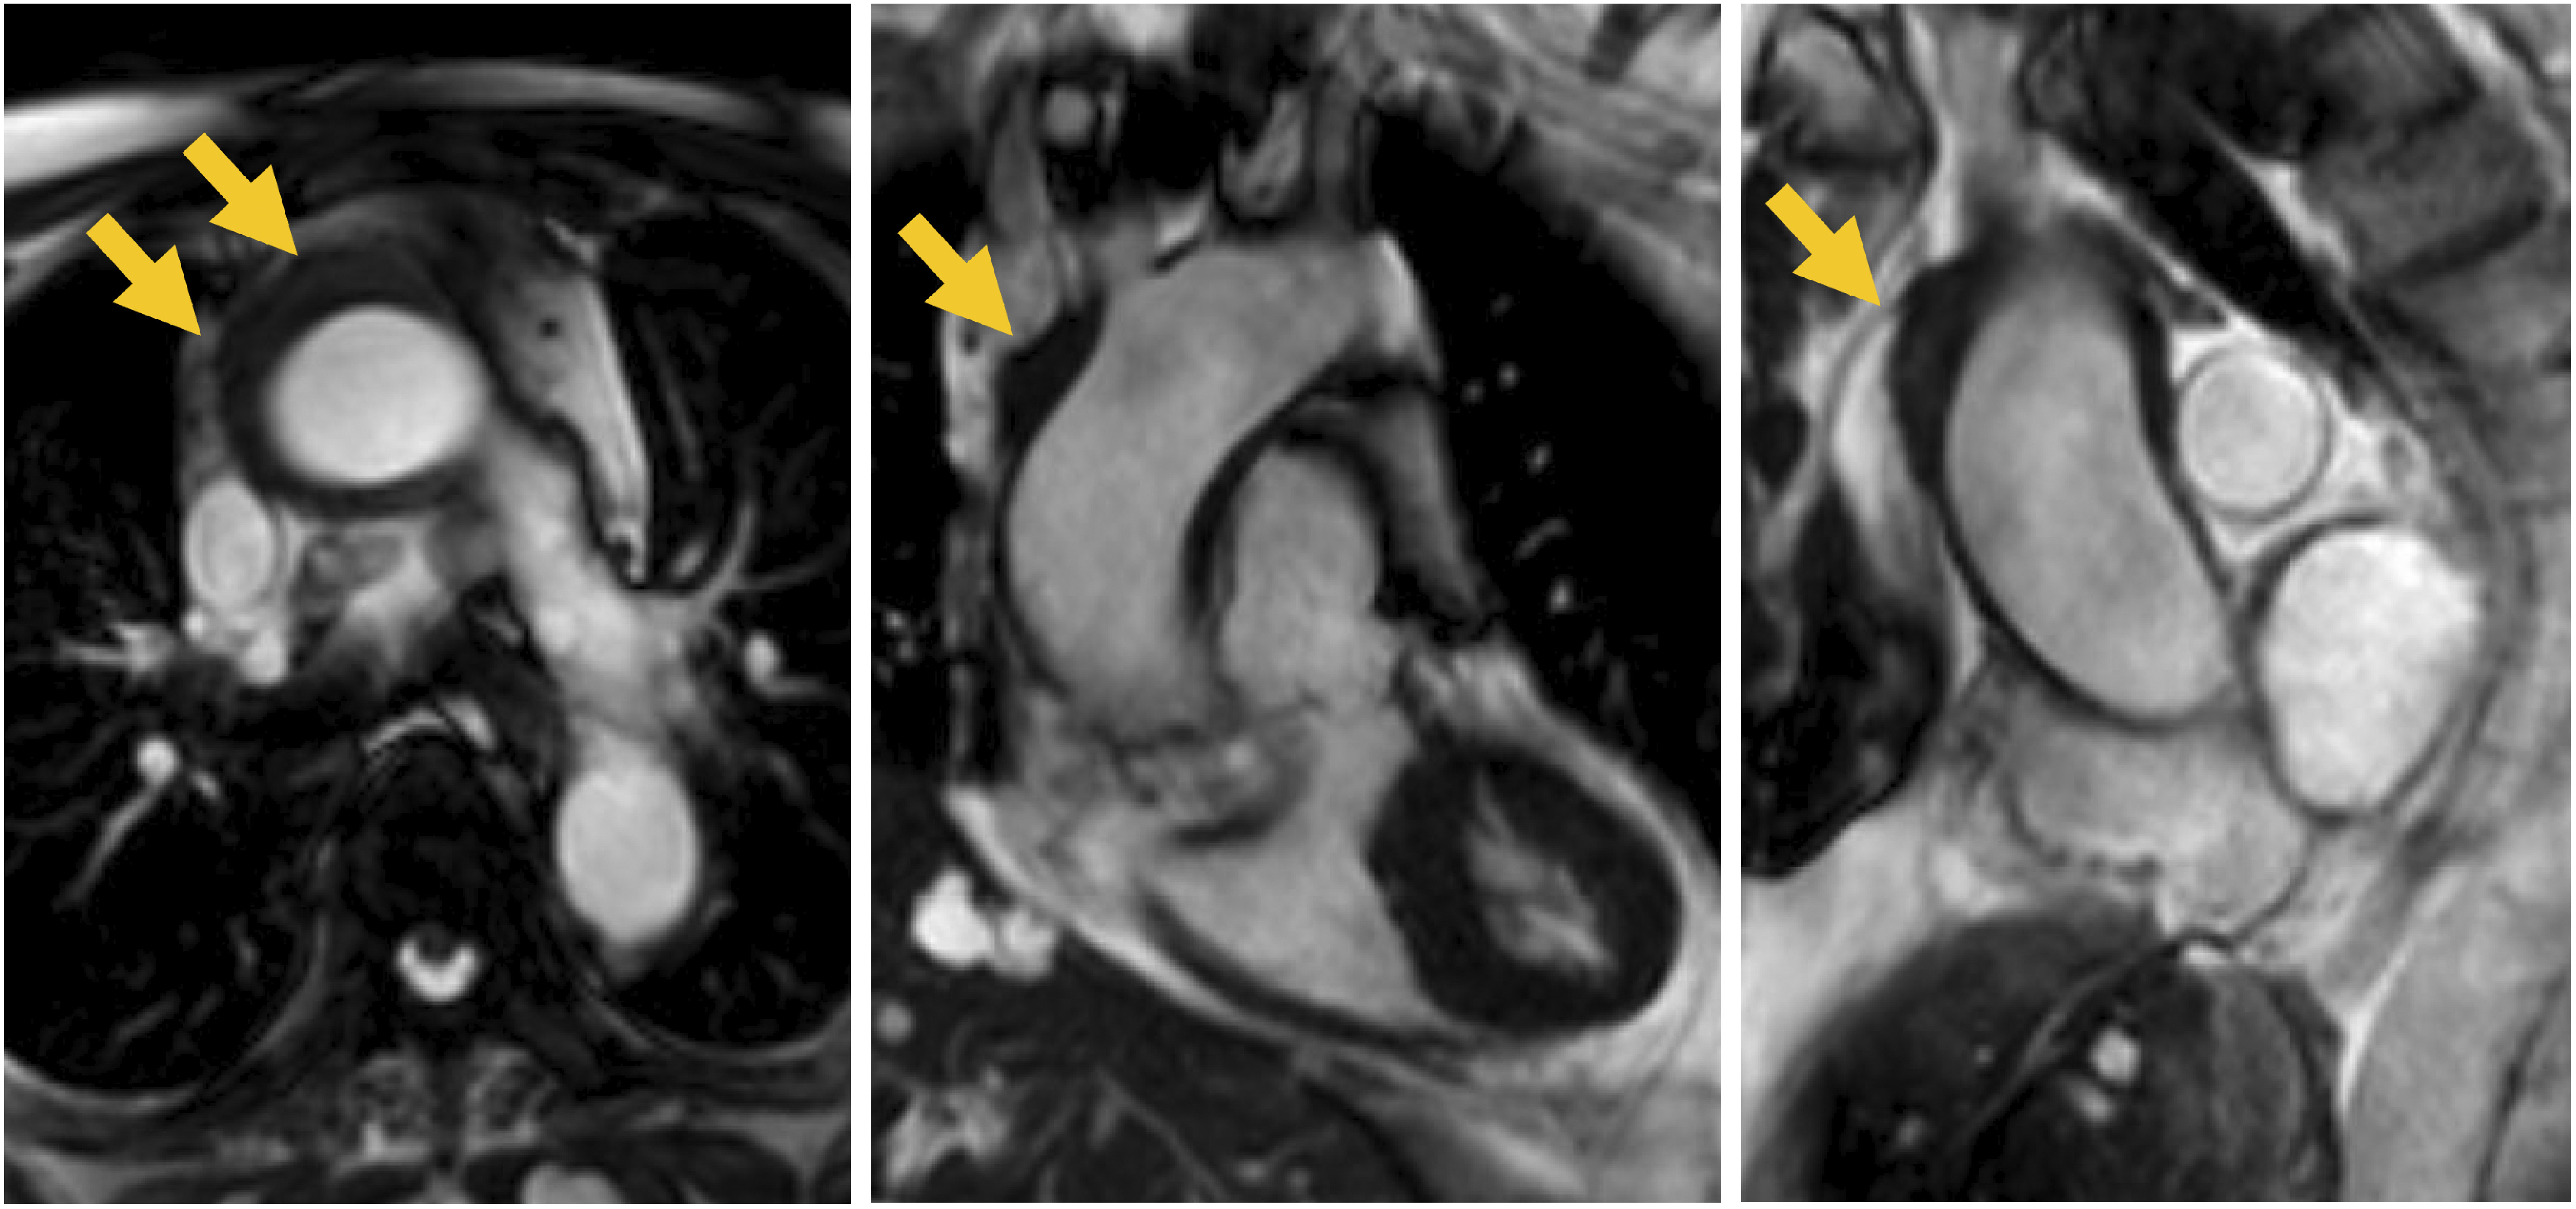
Fig. 2 T2-Weighted magnetic resonance imaging shows thickening of soft tissue around the ascending aorta.
